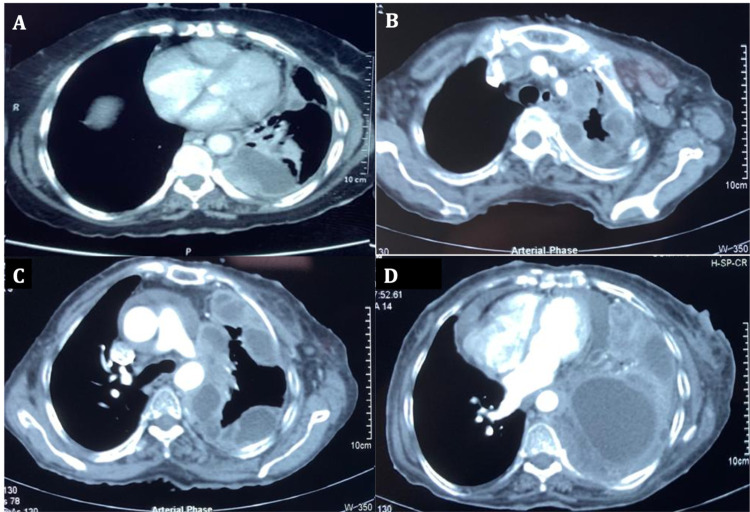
Chest CT A - CT chest performed six months prior showing left-sided minimal effusion with a pleural nodule; B, C, D - Current CT showing multiple loculations with a heterogeneous lesion involving the entire left hemithorax with mild pericardial effusion
